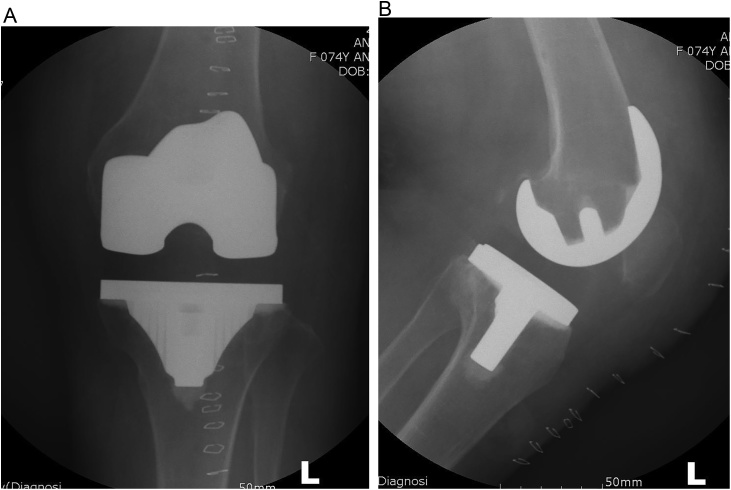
Immediate post-operative radiograph (A) Anteroposterior fluoroscopy showed well positioned implant and mediolateral symmetry (B) lateral fluoroscopy showed a patella on the front of femur that had not seen on the preoperative lateral radiography.
